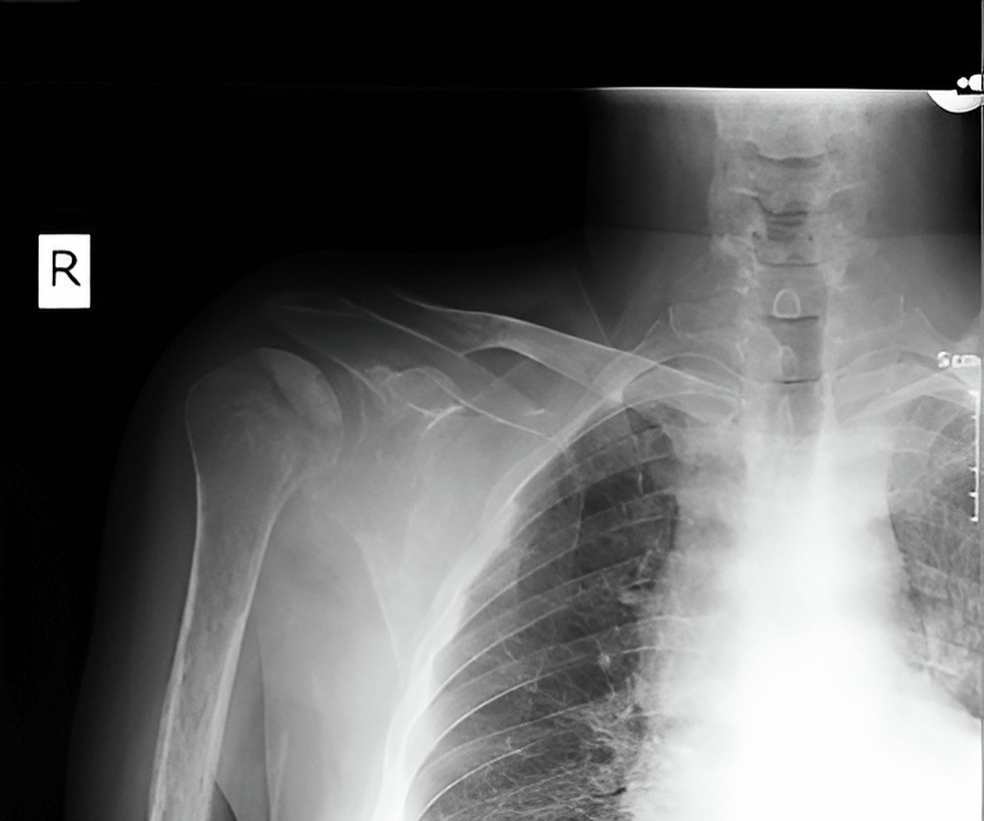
Preoperative X-ray image of the humerus head and scapula
